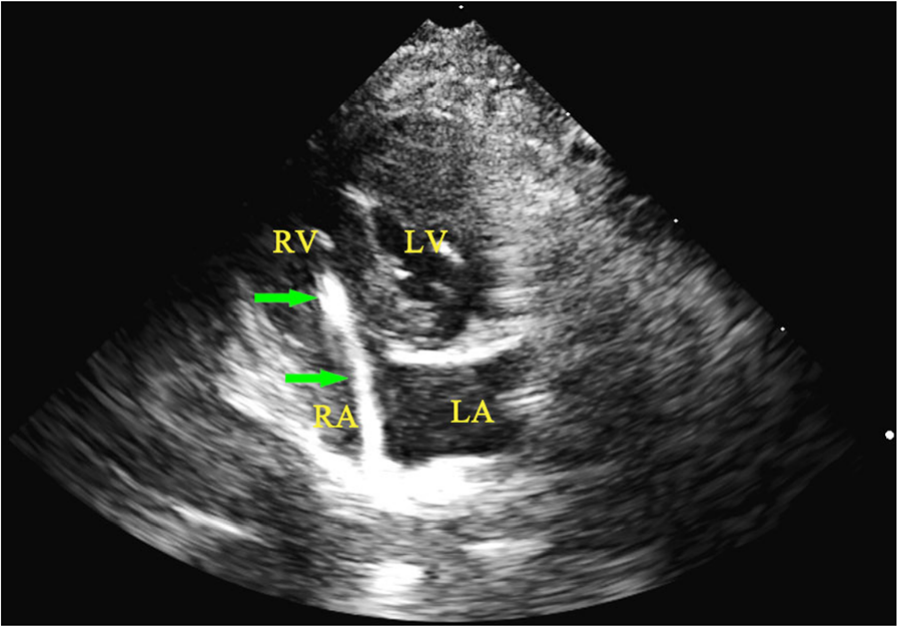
Confirmation the position of the catheter system by echocardiography. The position of catheter system, passing through the tricuspid valve, was confirmed by echocardiography in a four-chamber view. LA, left atrium; LV, left ventricle; RA, right atrium; RV, right ventricle.
